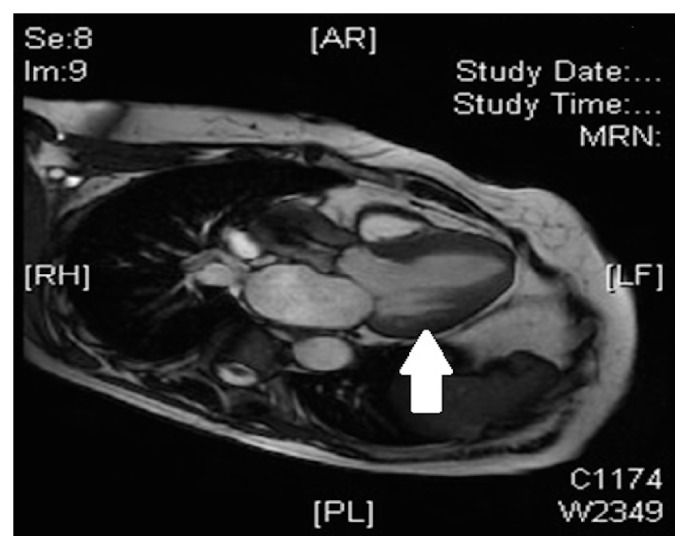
Gadolinium contrast cardiac magnetic resonance image showing left ventricular myocardial fibrosis in a patient with severe aortic stenosis and ventricular hypertrophy (arrow).
